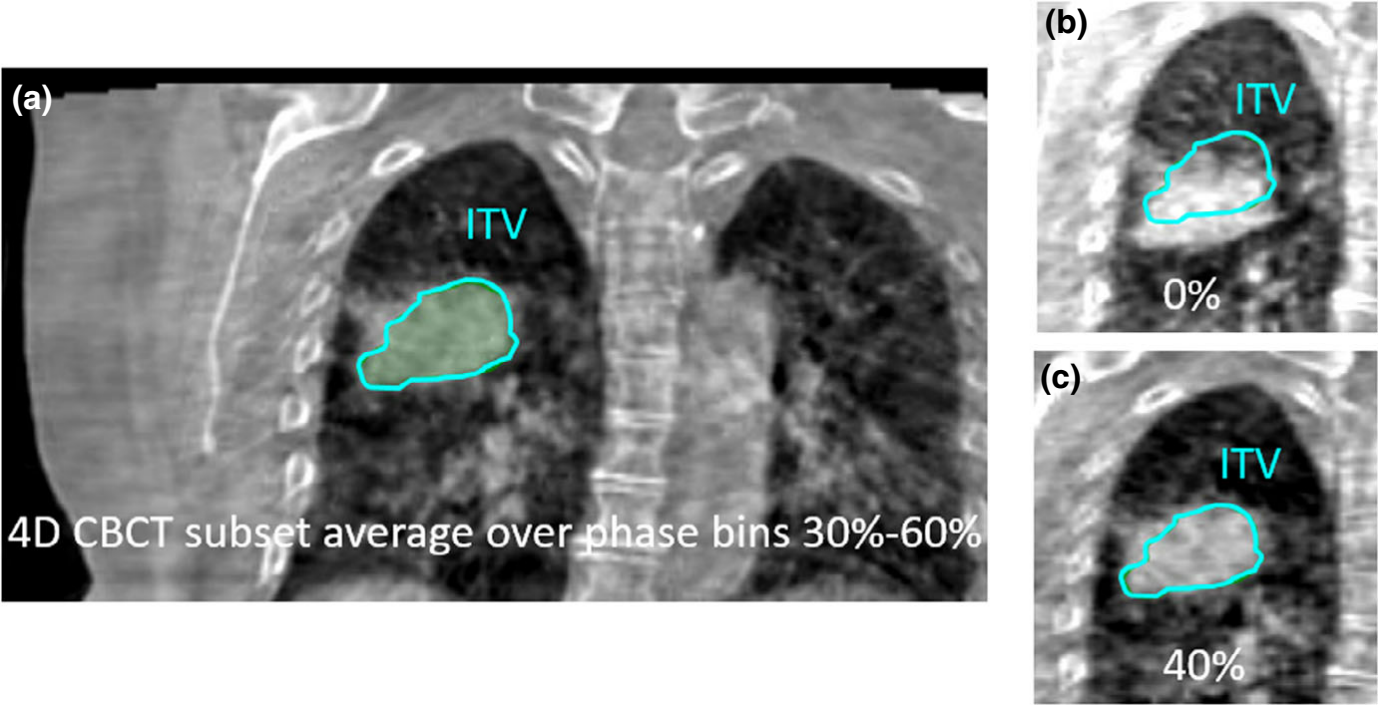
Coronal 4DCBCT images for the clinical lung case. (a) A 4DCBCT subset average over phase bins 30–60% is reconstructed for initial matching. (b) The 4DCBCT movie loop shows the target outside of the ITV in the 0% phase bin and (c) inside the ITV in the 40% phase bin, as expected.
